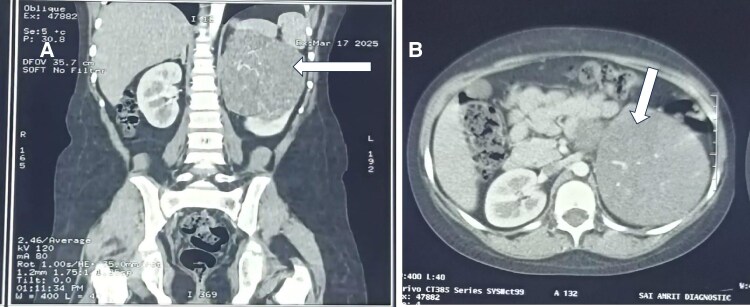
Contrast-enhanced computed tomography (CECT) abdomen demonstrating Wilms tumor. (A) Coronal section and (B) axial section reveals a well-defined large, solid, lobulated, heterodense, poorly enhancing, endo- and exophytic mass lesion measuring 11.6 × 10.2 × 10.7 cm in upper and mid-portions of the left kidney with no internal calcification or hemorrhage without involving the adrenal gland suggestive of Wilms tumor.
